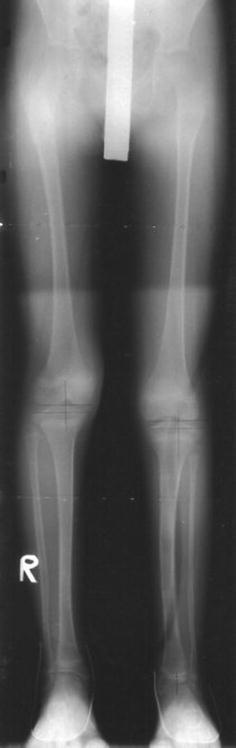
Radiograph of follow-up mechanical axis view
